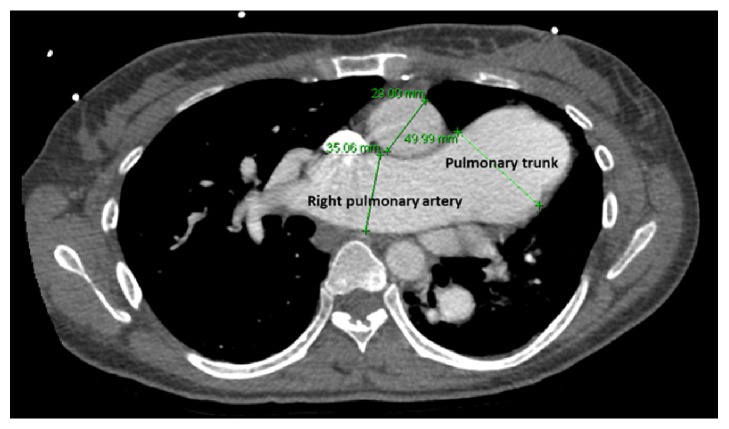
Computed tomographic angiography of the chest shows a markedly dilated pulmonary trunk, at 49.99 mm (normal < 30 mm); a pulmonary trunk-to-aorta ratio of 1.7 (normal < 1.0); and an enlarged right pulmonary artery, at 35.06 mm (normal < 20 mm).
